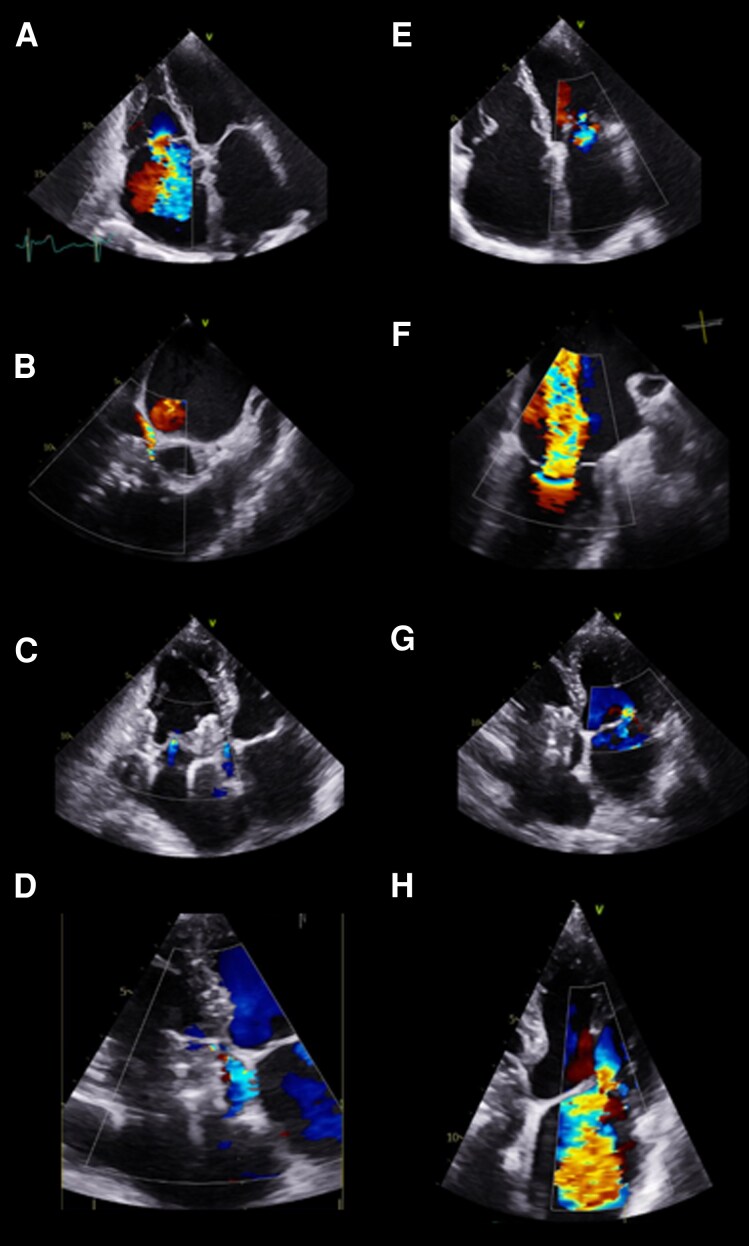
Transthoracic echocardiogram and transoesophageal echocardiography showing tricuspid regurgitation (a – TTE at baseline, B – TOE after TTVR implantation, C – TTE at discharge, D – TTE two months after TTVR) and mitral regurgitation (E – TTE at baseline, F – TOE after TTVR implantation, G – TTE at discharge, H – TTE two months after TTVR).
